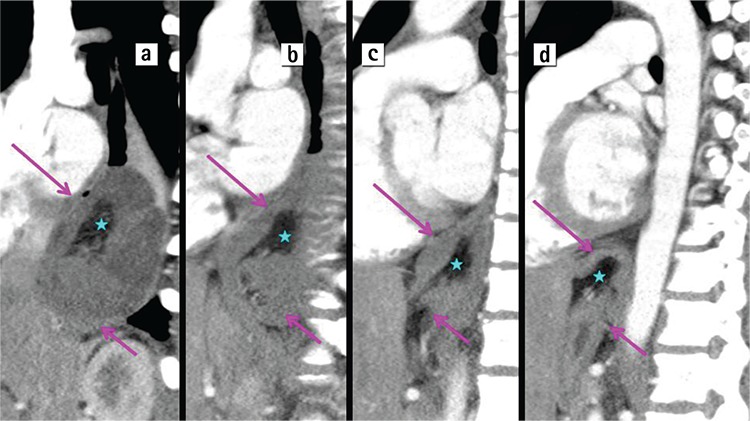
a-d. Sagittal view, (pink arrows show stomach layers, blue stars omental fat). Contrast-enhanced computed tomography showed all layers of the stomach and omental fat herniated into the lower oesophagus through a hiatal hernia.
